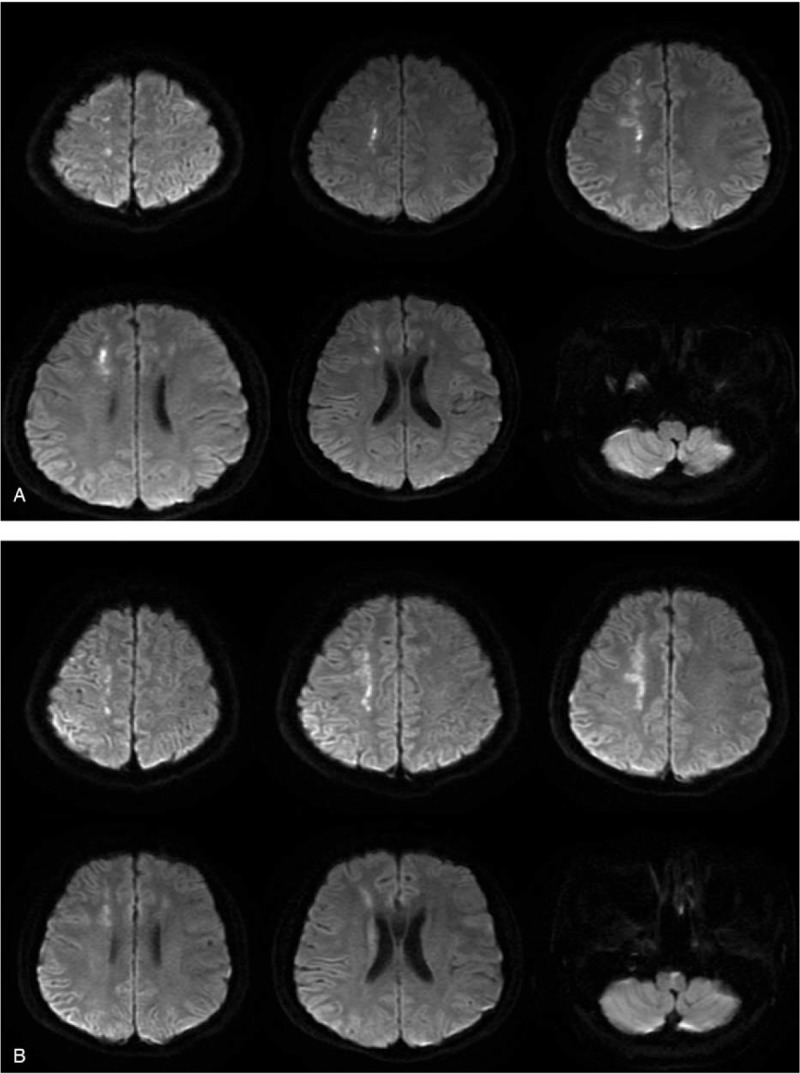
MRI indicated (A) hyper-intense DWI signals of the central lesion in the right centrum and (B) hyperintense DWI signals of lesions in the bilateral centrum semiovale and right lateral ventricle with slightly elevated DWI signals of lesions in the pons.
